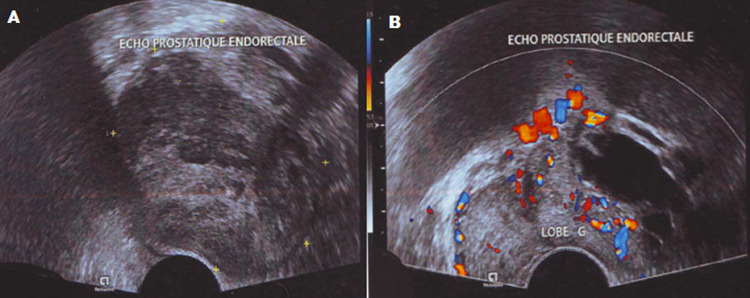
échographie endorectale en mode B (a) et Doppler couleur; (b) formation tumorale hétérogène vascularisée au Doppler couleur du lobe gauche de la prostatique
